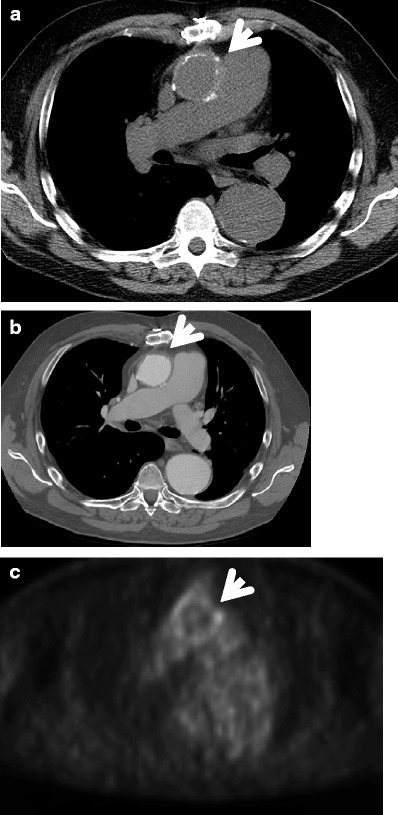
A 67-year-old female several months after ascending aneurysm repair, with low-grade fever and malaise. Axial non-contrast-enhanced (a) and contrast-enhanced (b) images of the chest demonstrate an ascending aortic graft with surrounding minimal aortic wall thickening (*arrowheads* in a and b respectively). The area was shown to be positive for moderate 18-FDG uptake on the PET-CT image (*arrowhead* in c). Blood cultures revealed *Staphylococcus aureus* infection, concerning for infectious aortitis
